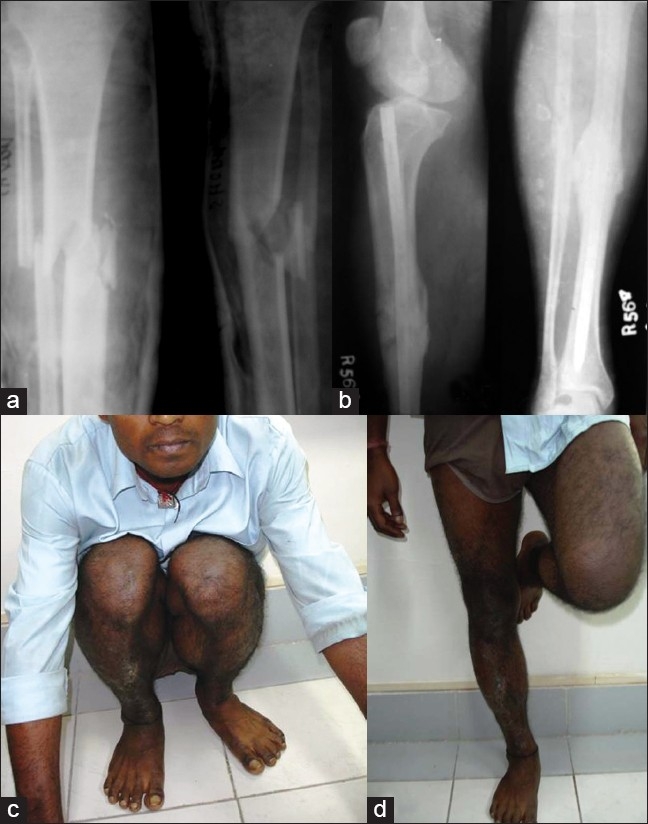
(a) Pre-operative X-rays showing diaphyseal fracture of middle third of tibia and fibula. (b) Follow up X-rays showing expandable nail in place and fracture consolidation. (c,d) Clinical function at final follow up
